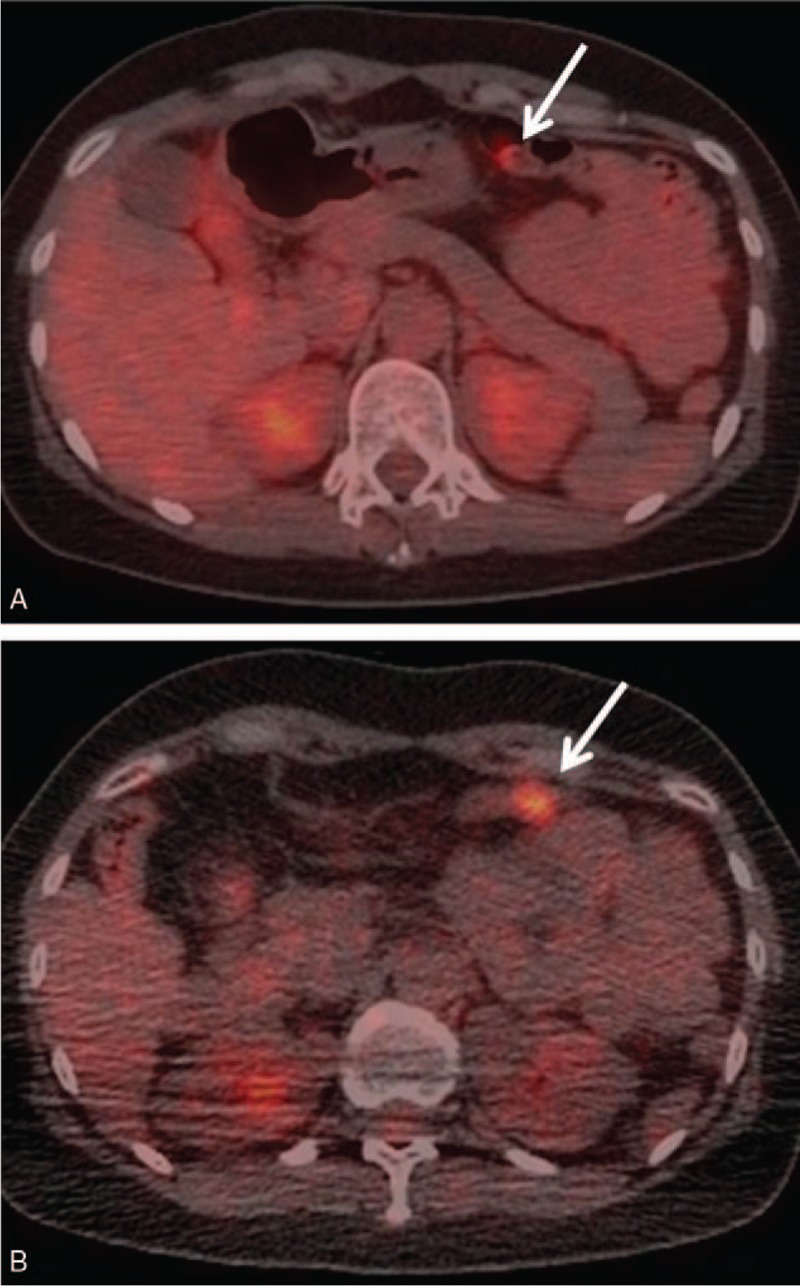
Axial [^18^F]-fluorodeoxyglucose (FDG)-positron emission tomography/computed tomography images of a 48-year-old woman. On the initial-time-point image, FDG uptake (maximum standard uptake value [SUV max]: 13.6) in the transverse colon was ambiguous and difficult to distinguish from the background or physiological uptake (arrow) (A). Nodular uptake (SUV max: 8.4) was definitive on a delayed-time-point image (arrow) (B). The lesion was pathologically proven to be adenocarcinoma.
